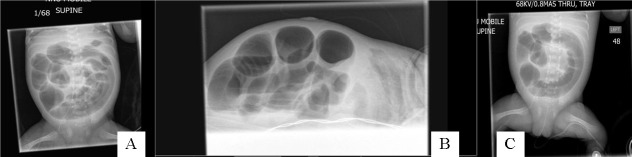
Figure 2: (A): AXR of Case 2 on day 44 of life, showing dilated bowel loop surrounding a cyst like appearance in the central abdomen; (B): Lateral shoot through x-ray of the abdomen of case 2 on day 44 of life showing dilated bowel in the central abdomen. No free air is seen; (C): AXR of Case 2 on day 46 of life, showing fixed dilated bowel loop surrounding a cyst like appearance in the central abdomen.
